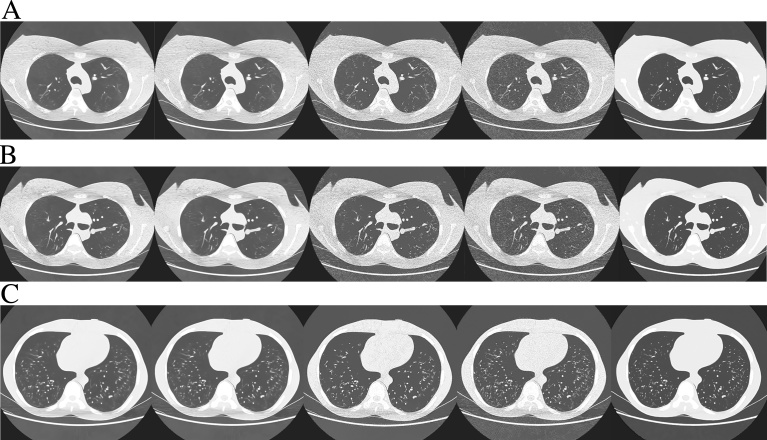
Testing ULDCT images denoised with CAE, LNLM, and BM3D. A, B, and C show the upper-, middle-, and lower-lung fields, respectively. The denoising methods and parameters of these images were: 1st column, BM3D with the parameter of sigma = 25; 2nd column, BM3D with the parameter of sigma = 20; 3rd column, LNLM with the parameter of h = 0.03; 4th column, LNLM with the parameter of h = 0.02; 5th column, CAE. Abbreviation: CAE, convolutional auto-encoder; ULDCT, ultra-low-dose CT; LNLM, large-scale nonlocal means.
